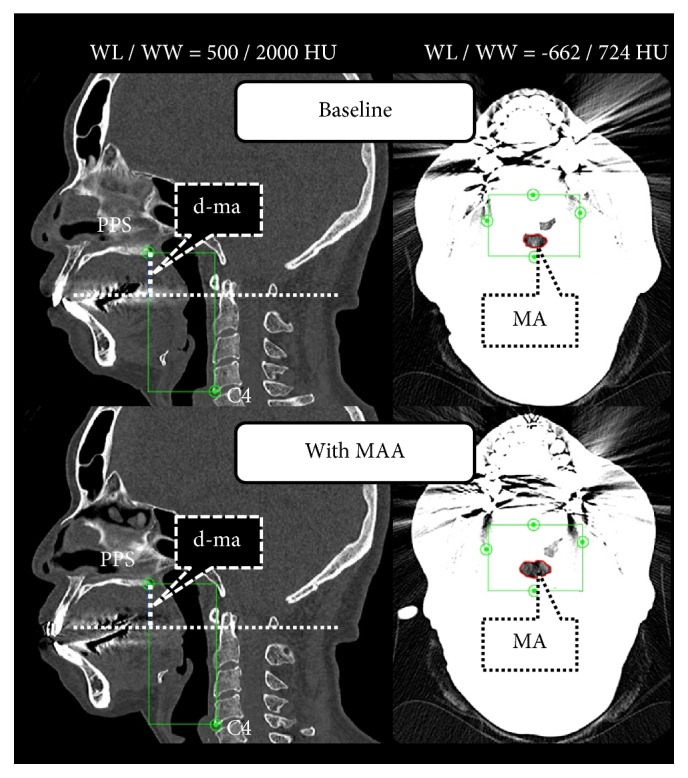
The minimum area (MA) on multiplanar reconstruction (MPR) images. The slice datasets were coordinated as follows: (1) The median sagittal cross-sectional image was set by using the baseline (T1) data (window level/window width [WL/WW] was set to the CT-bone value of 500/2000 HU). (2) The craniocaudal direction was set with the upper end as the palatal pharyngeal space (PPS) and the lower end as the lowermost anterior point of the fourth cervical vertebrae (C4) on the MPR image by verifying on the median sagittal, axial, and coronal cross-sectional images. (3) The WL/WW was set to the airway component value of -662/724 HU, the site where the cross-sectional area within that range was the narrowest by verifying on the axial cross-sectional image was set as the MA, and the anteroposterior diameter, width, and cross-sectional area were measured. (4) The WL/WW was again set to the CT-bone value of 500/2000 HU, and the vertical distance (d-ma) from the PPS to the MA was measured on the median sagittal cross-sectional image. (5) The median sagittal cross-sectional image was set using data with the mandibular advancement appliance (MAA), and each d-ma obtained from the baseline data was reproduced as multislice computed tomography (MSCT) data with MAA. (6) The WL/WW was set to the airway component value of -662/724 HU, and the anteroposterior diameter, width, and cross-sectional area were measured by verifying on the axial cross-sectional image.
